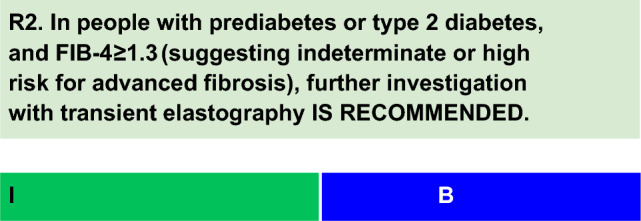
Quantification of fibrosis and steatosis can be performed using liver elastography [22]. Elastography can distinguish the following stages of fibrosis: absent or mild (F0/F1), moderate (F2), advanced (F3), and cirrhosis (F4). Among ultrasound elastography methods, transient elastography (FibroScan^®^) is currently the most validated noninvasive technology [22].Sequential combinations of markers with a lower cutoff to rule out advanced fibrosis and a higher cutoff to rule out cirrhosis can reduce the false negative rate for advanced fibrosis. According to an individual patient data meta-analysis of 37 studies (n = 5,735; 33% with T2D), the sequential combination of FIB-4 (cutoffs: < 1.3; ≥ 2.67) and liver stiffness measurement by transient elastography (cutoffs: < 8.0 kPa; ≥ 10.0 kPa) had a sensitivity and specificity of 66% and 86%, respectively. This strategy resulted in a false negative rate of 9% for advanced fibrosis [23]. Upper cutoffs to rule-in cirrhosis (e.g., 12.0 kPa) could lead to a further decrease in the need for liver biopsies.Magnetic resonance elastography (MRE) has good accuracy in quantifying liver fat and assessing fibrosis. However, the high cost and low availability of these methods are limitations [24].The proper selection of methods for assessing fibrosis requires consideration of local availability. Noninvasive methods for examining MASH are not yet available.
